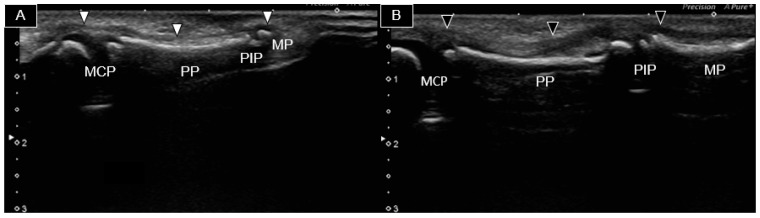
Ultrasound image of the palmar side of the fourth right (A) and left (B) fingers. The white arrowhead indicates the right finger flexor tendon, whereas the black arrowhead indicates the left flexor tendon. MCP, metacarpophalangeal joint; PP, proximal phalanx; PIP, proximal phalangeal joint; MP, middle phalanx.
